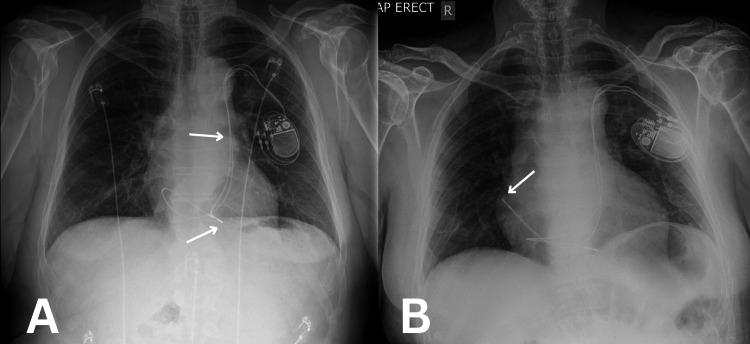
Post-procedure chest X-rays. A. Post permanent pacemaker (PPM) implant anteroposterior (AP) chest X-ray shows evidence of left persistent superior vena cava (LPSVC) due to the trajectory of the PPM leading down to the right atrium and ventricle (top arrow). The bottom arrow points to the displaced right atrium lead. B. Post-lead repositioning AP chest X-ray shows a successful right atrium lead positioning (arrow).
